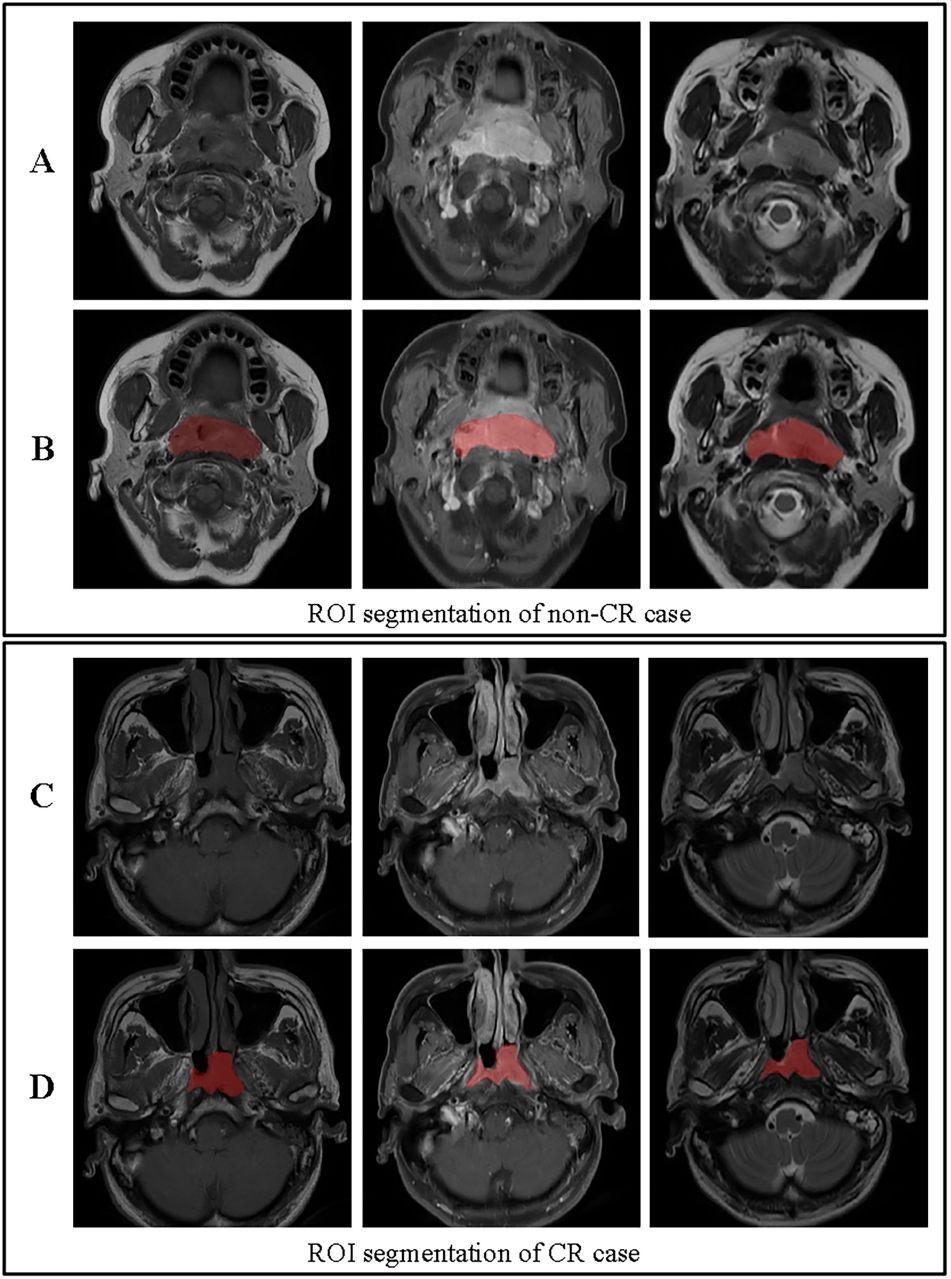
ROI segmentation for non-CR and CR cases. (A) Original images for a non-CR case, from left to right: T1, contrast-enhanced T1-weighted, and T2 sequences. (B) ROI segmentation for the non-CR case on T1, contrast-enhanced T1-weighted, and T2 images. (C) Original images for a CR case, from left to right: T1, contrast-enhanced T1-weighted, and T2 sequences. (D) ROI segmentation for the CR case on T1, contrast-enhanced T1-weighted, and T2 images. This figure demonstrates the differences in ROI segmentation between non-CR and CR cases, highlighting the segmented tumor regions in red.
